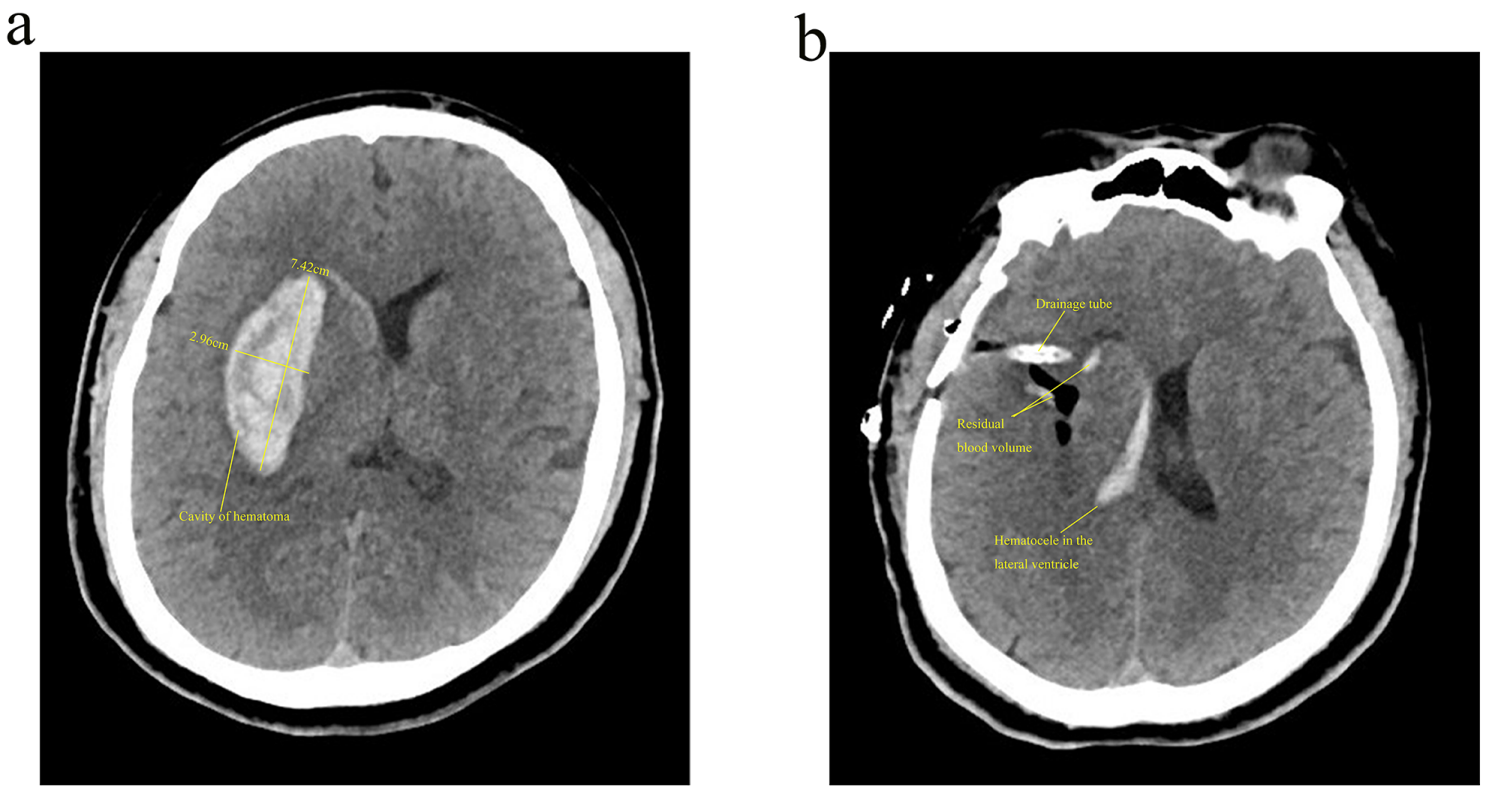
A 56-year-old woman experienced right hemiplegia for 3.5 h and underwent emergency neuroendoscopic removal of cerebral hematoma. (a) Preoperative CT, hematoma volume 36.724 ml; (b) Postoperative CT, remaining hematoma volume 2.031 ml
